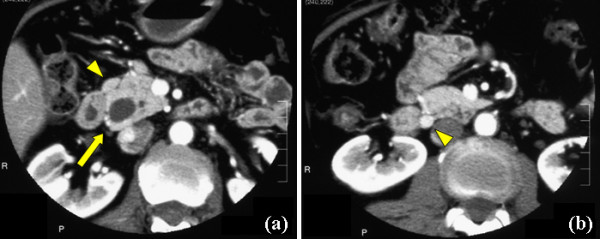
Contrast-enhanced CT shows the markedly dilated CBD and 2 enlarged lymph nodes in the peripancreatic region. (a) The marked dilated CBD (arrow) and one of 2 enlarged lymph nodes near the upper border of the pancreas (arrow head) are detected. (b) Another enlarged lymph node near the lower border of the pancreas (arrow head) is found.
